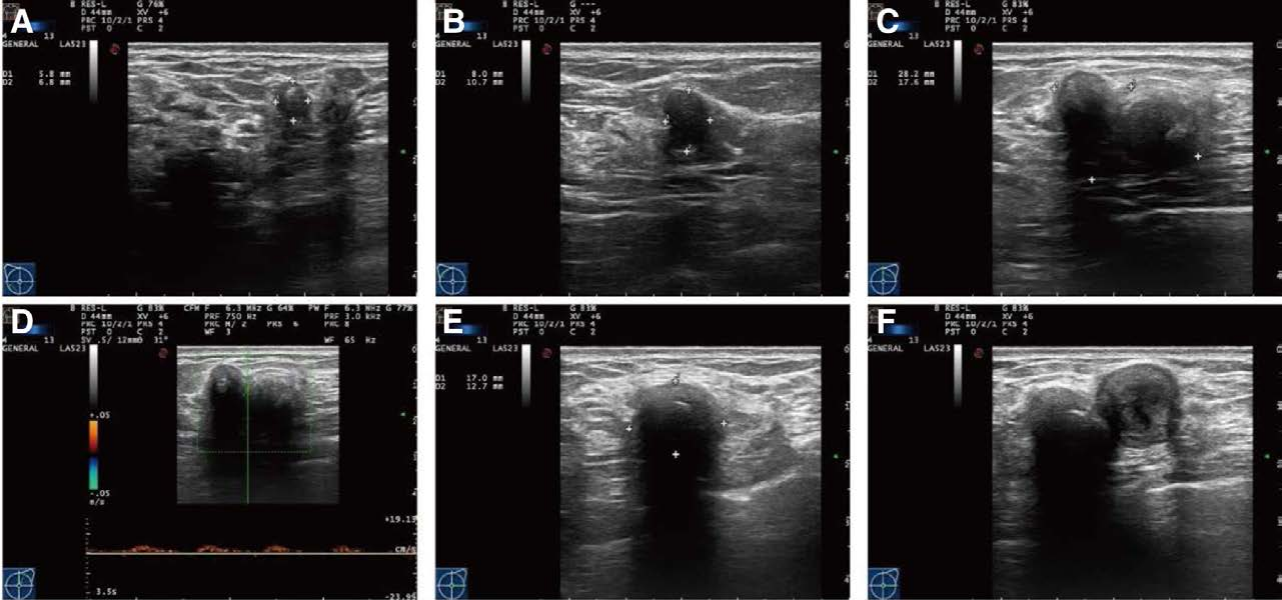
Results of bilateral breast color ultrasound examination: (A) A right breast nodule measuring 0.6 × 0.7 cm at the 8 o’clock position, 1.9 cm away from the nipple, which was straight and had a thick light spot with uneven internal echo. The nodule was assessed as BI-RADS 4B. (B) A right breast nodule measuring 0.8 × 1.1 cm at the glandular edge at 10 o’clock, which was assessed as BI-RADS 4B. (C, D) There was a 2.8 × 1.8 cm nodule at 1 o’clock in the left breast, 3.8 cm away from the nipple, with irregular shape and unclear boundary, which was assessed as BI-RADS 4C. CDFI indicated that blood flow signals could be seen in the nodule. (E, F) A 1.7 × 1.3 cm erect hypoechoic nodule was at the 3 o’clock position in the left breast with a strong echo light spot, which was assessed as BI-RADS 4B. There seemed to be another vertical nodule fusion beside it measuring 1.3 × 1.5 cm with uneven echo, which was BI-RADS 4A. CDFI = color doppler flow imaging.
